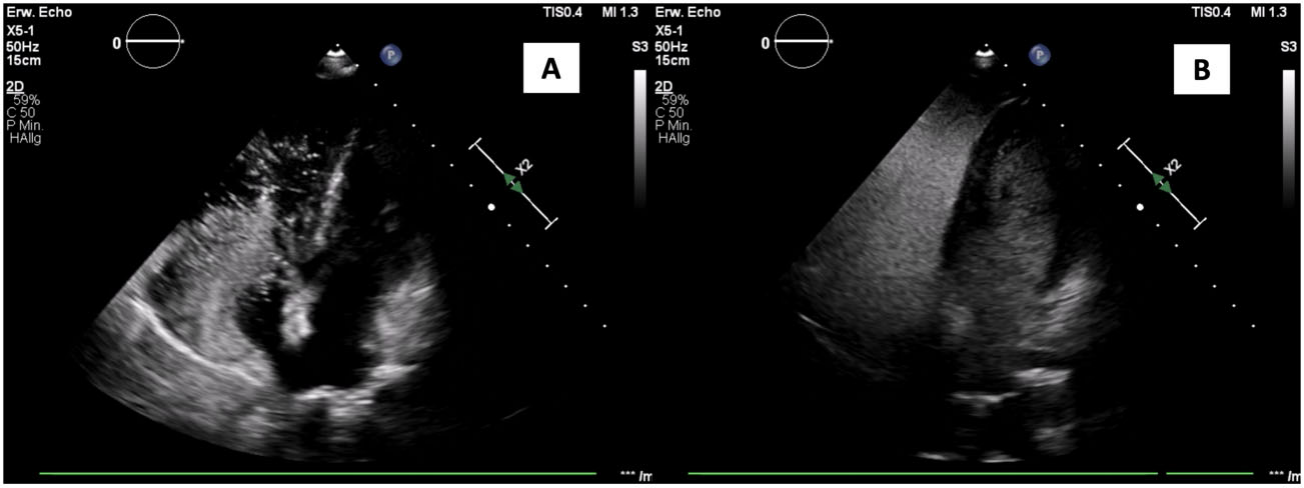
Transthoracic echocardiography with peripheral injection of non-transpulmonary contrast agent. Persistent foramen ovale closure device *in situ* without residual (early) interatrial shunt (*A*). Delayed appearance of microbubbles in the left atrium and ventricle after three to four heart beats (*B*) indicating massive intrapulmonary shunting.
